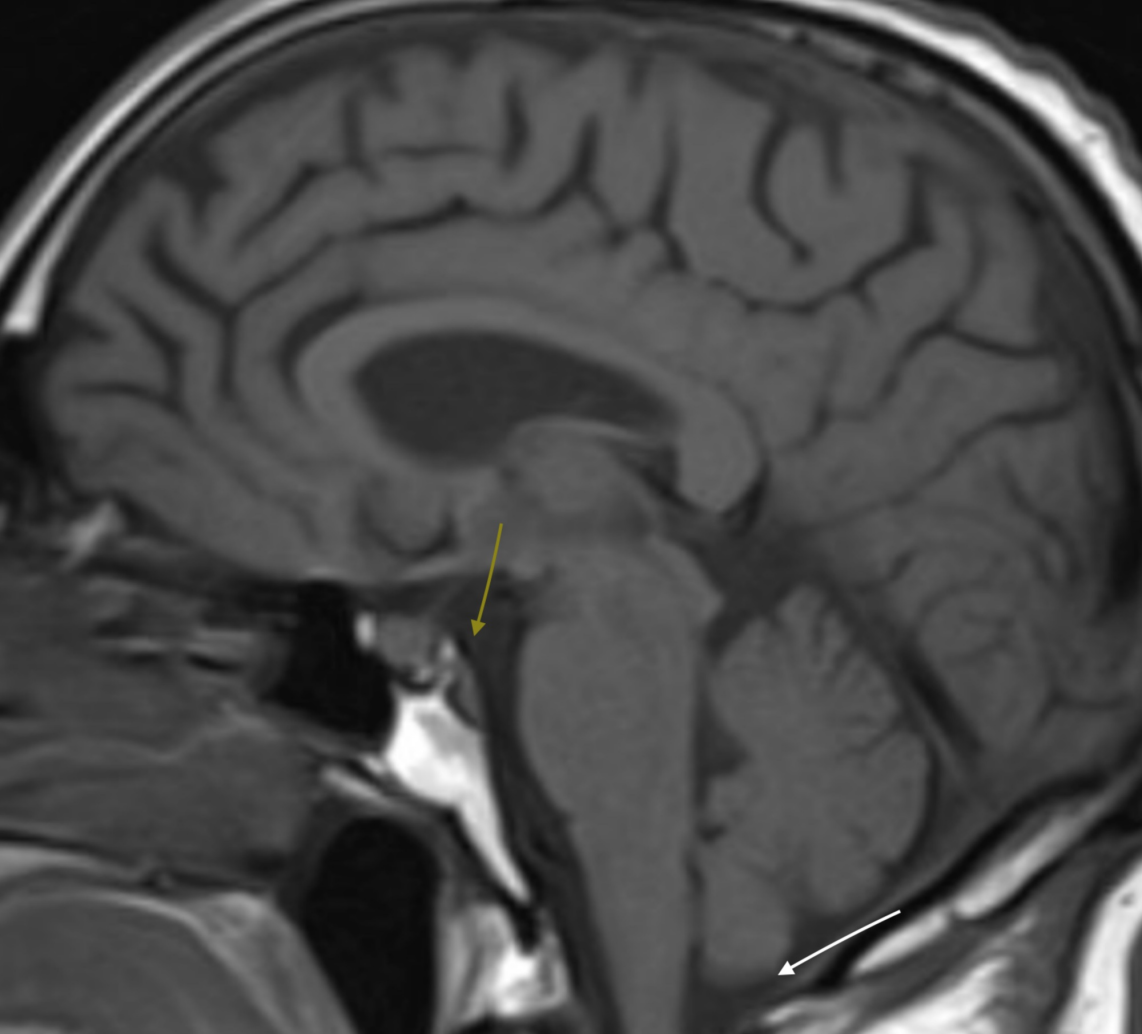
T1 sagittal MRI demonstrating capacious foramen magnum (white arrow) with generous basi-cranial flexion with absence of basilar impression (yellow arrow) MRI: magnetic resonance imaging
